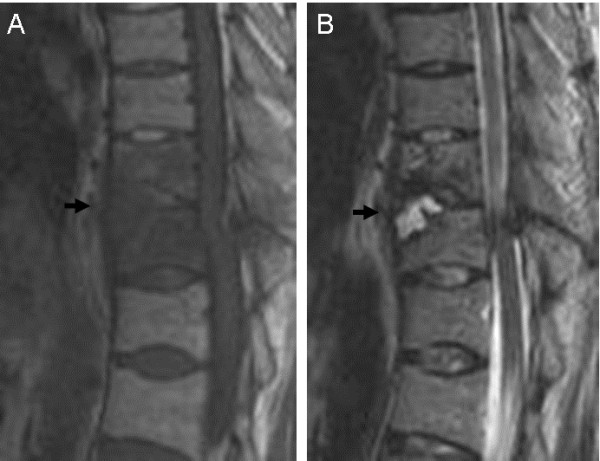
Preoperative magnetic resonance imaging of a patient with Parkinson’s disease with a thoracic spine fracture in a severely ankylosed spine. The fracture site (T11: arrow) shows low intensity on the T1-weighted sagittal image (A) and high intensity on the T2-weighted sagittal image (B), suggesting the presence of fluid accumulation.
